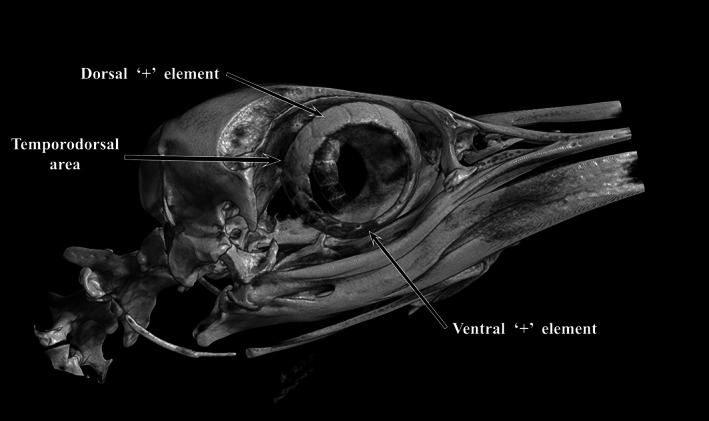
Micro‐CT image of the whole head of a king penguin (*Aptenodytes patagonicus*, K1) showing a dorsal ‘+’ element at 12 o'clock. The temporo‐dorsal area was the widest part of the scleral ring. It was also apparent that the ventral ‘+’ element was further rostral than the dorsal ‘+’ element
